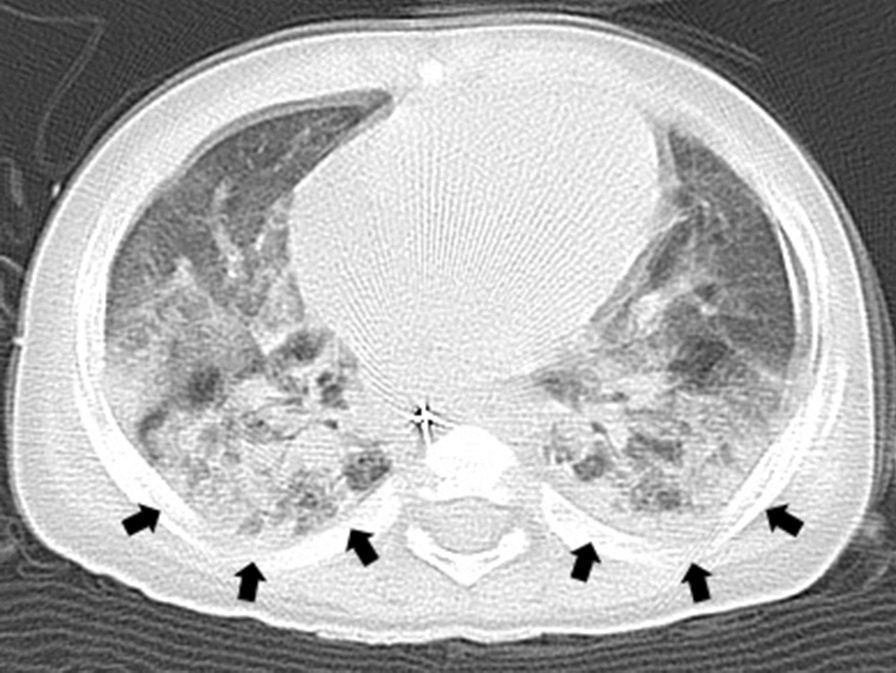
Computed tomography of the lung on postoperative day 1 showing bilateral ground-glass opacities (black arrow)
